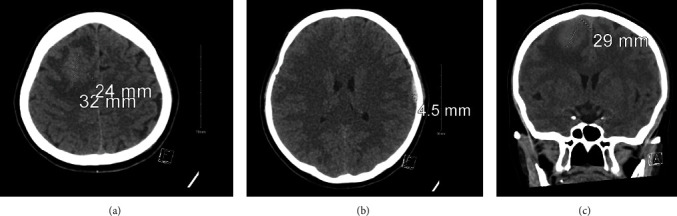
(a, b) CT brain axial view and (c) CT brain coronal view. (a, c) Right frontal subcortical hyperdensity with vasogenic edema and mass effect measuring 3.2 × 2.4 suggests a parenchymal hemorrhage. (b) Left temporal bone nondisplaced fracture with associated small epidural hematoma and periorbital fat stranding.
